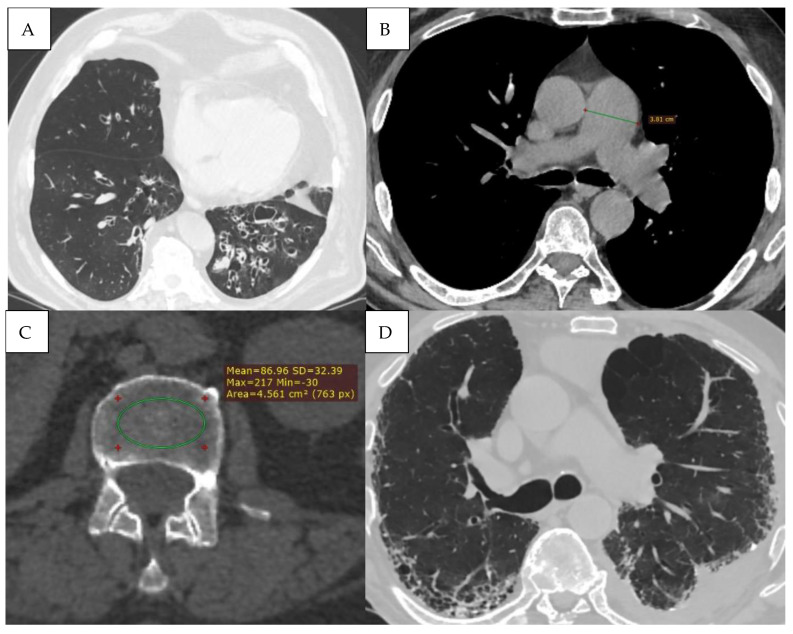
(A) Bronchiectasis (multiple types of bronchiectasis in the lower lobe of the right lung, some with mucoid impaction). (B) CT signs of pulmonary hypertension (diameter of the pulmonary trunk was 3.8 cm (normal < 2.9 cm)). (C) Osteoporosis, bone density measurement at the level of L3 (86.96 HU). (D) ILD (Interstitial Lung Disease), subpleural honeycombing formed by clustered cysts stacked together in several layers. Reticular pattern. Left pleural effusion.
